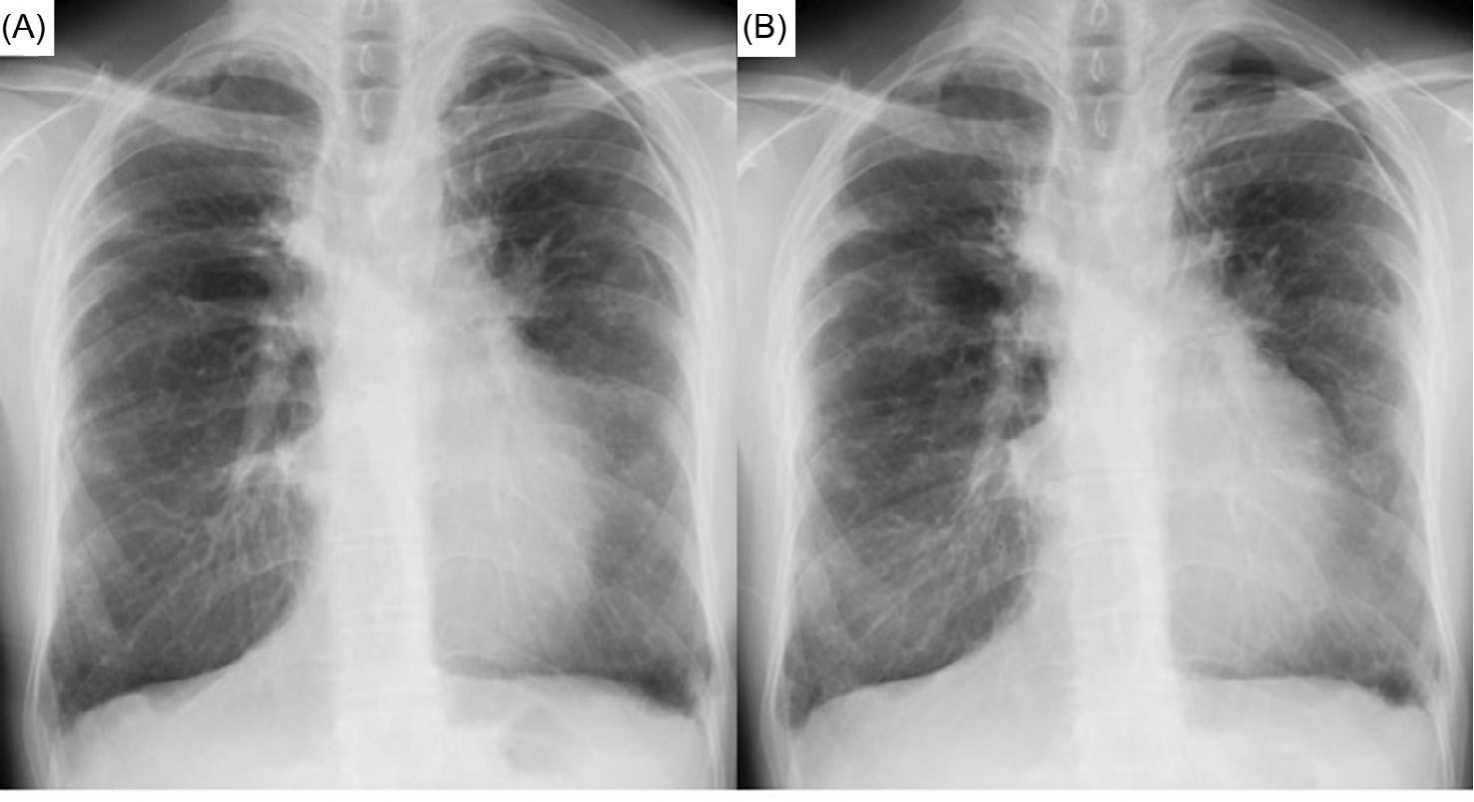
Chest radiography reveals pneumothorax of the left lung at 1 year before presentation (A) and recurrent pneumothorax in the left lung and an infiltrative shadow outside both lungs (B).
